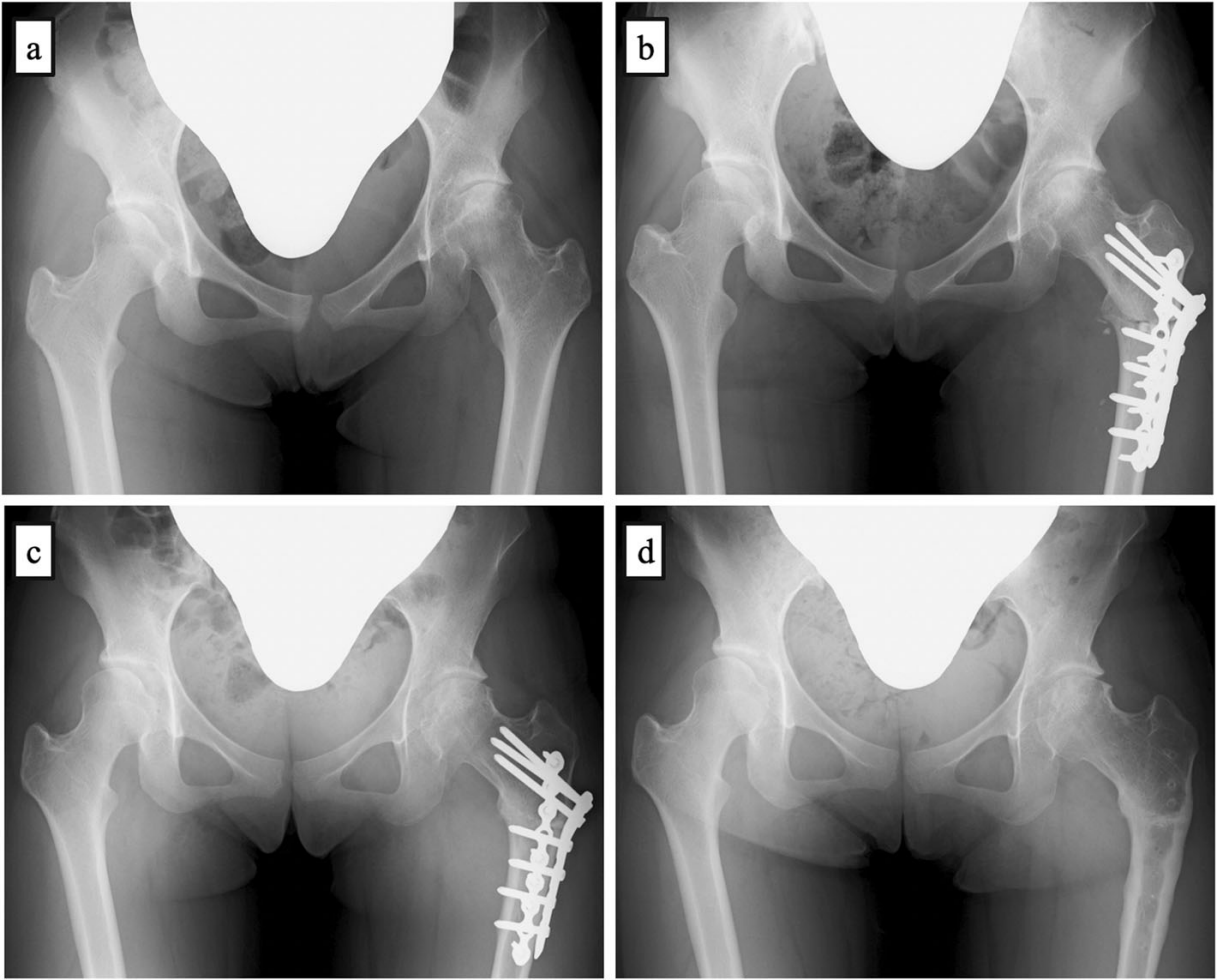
Radiography AP view (a: preoperation, b: postoperation, c: 1 year after surgery, d: 1.5 years after surgery). a: Irregularity of the left anterolateral femoral head and a subcartilaginous cyst are seen. Lateral center-edge, Tonnis, and Sharp’s angles (lt/rt) were 25/25, 10/10, and 45/45 degrees, respectively. There were no signs of femoroacetabular impingement. b: Immediately after surgery. c: 1 year after surgery. Joint space narrowing and femoral head osteosclerosis improved, and there was improved hip conformity. d: 1.5 years after surgery. Implant removal
